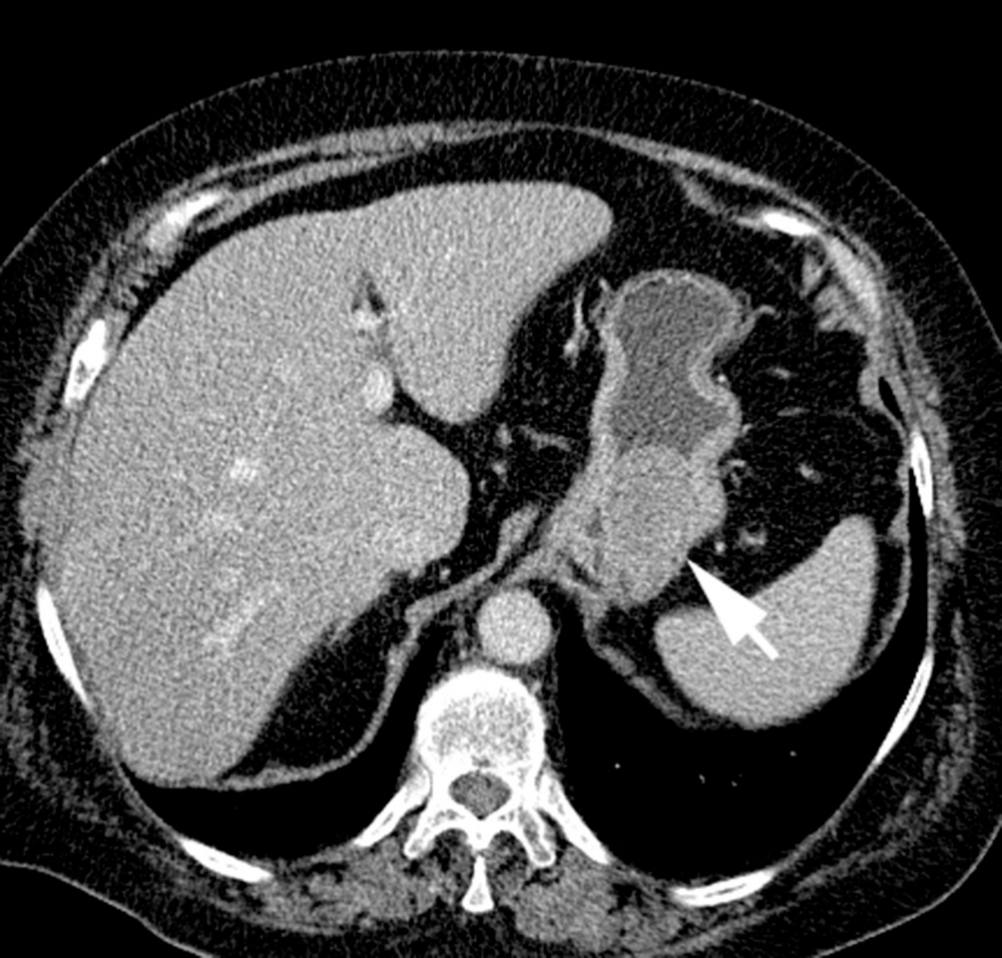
A nodular, contrast-enhancing gastric tumour, about 4 cm in diameter, is seen on the CT scan (arrow).
